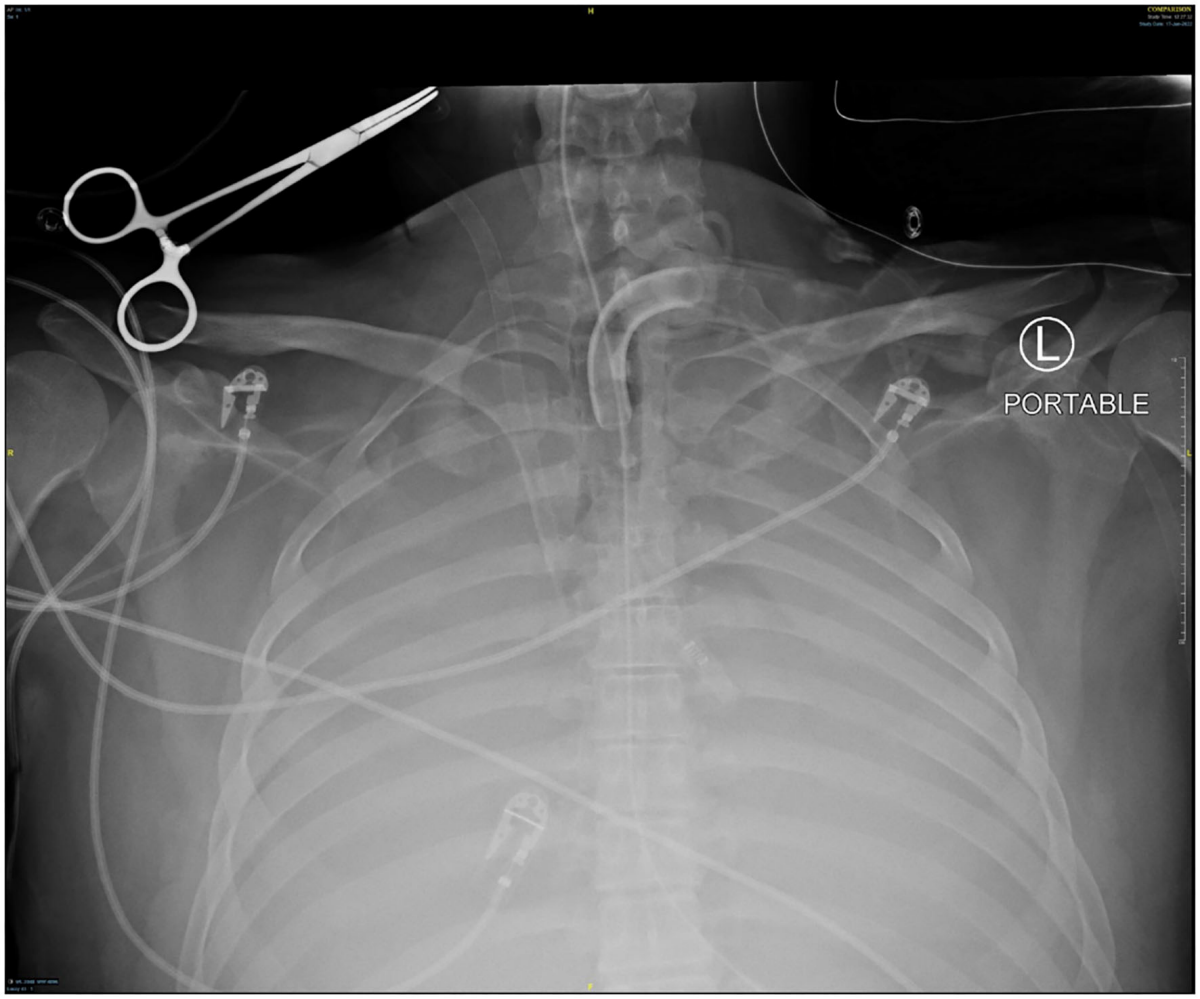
Chest x-ray off ventilator support.
